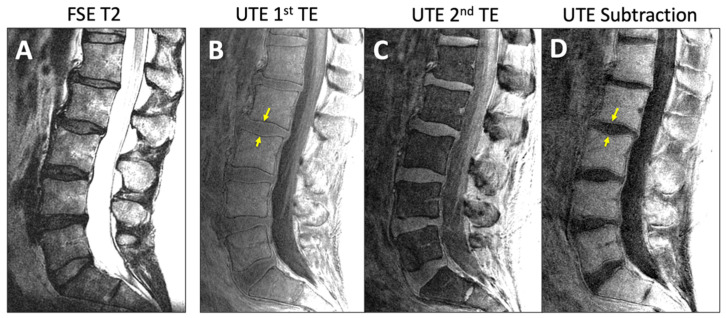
Representative sagittal MR images of lumbar spine of an asymptomatic subject acquired with (A) conventional fast spin echo T2 (FSE T2) and (B–D) ultrashort echo time (UTE) sequences. UTE MR images from (B) 1st TE minus (C) 2nd TE yielded (D) the subtraction image. Yellow arrows indicate the DVJ.
